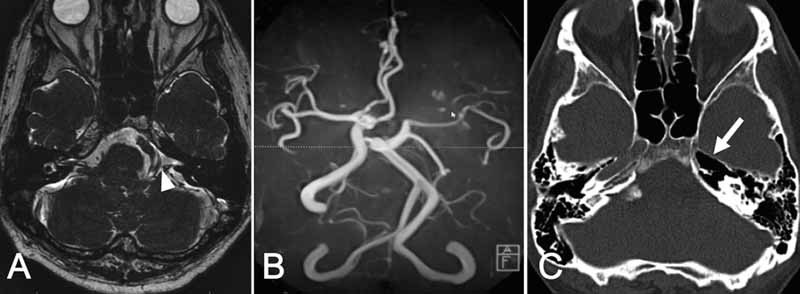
Preoperative neuroradiological imaging. (A) Heavy T2-weighted magnetic resonance imaging. The root exit zone of the left facial nerve was compressed by the tortuous vertebral artery (arrowhead). (B) Magnetic resonance angiography shows absence of the left internal carotid artery. The left middle cerebral artery is fed from the posterior circulation via the posterior communicating artery. The left vertebral artery is large and ectatic. (C) Computed tomography bone window image demonstrating agenesis of the left carotid canal (arrow).
